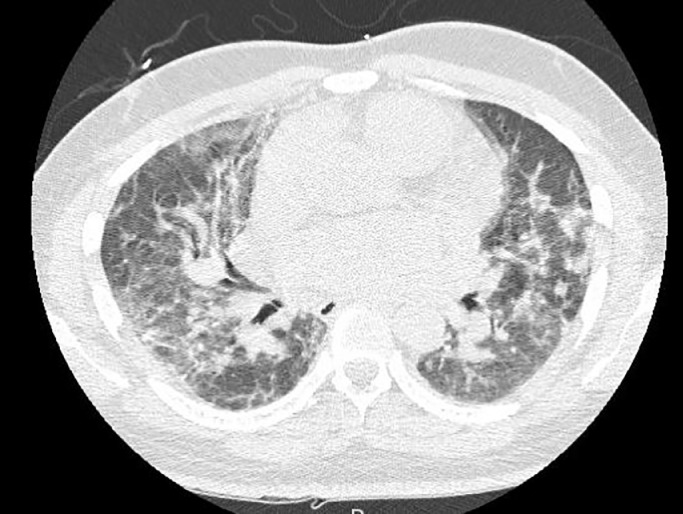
Computed tomography scan of the chest consistent with severe interstitial lung disease.
